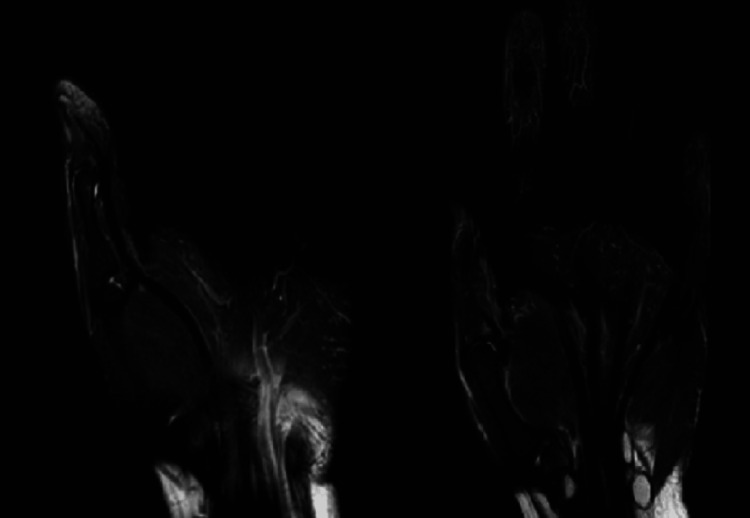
Coronal T2 magnetic resonance imaging (MRI) of the right hand demonstrating mildly increased signal at the base of the thumb proximal phalanx, but no overt evidence of FPL rupture
